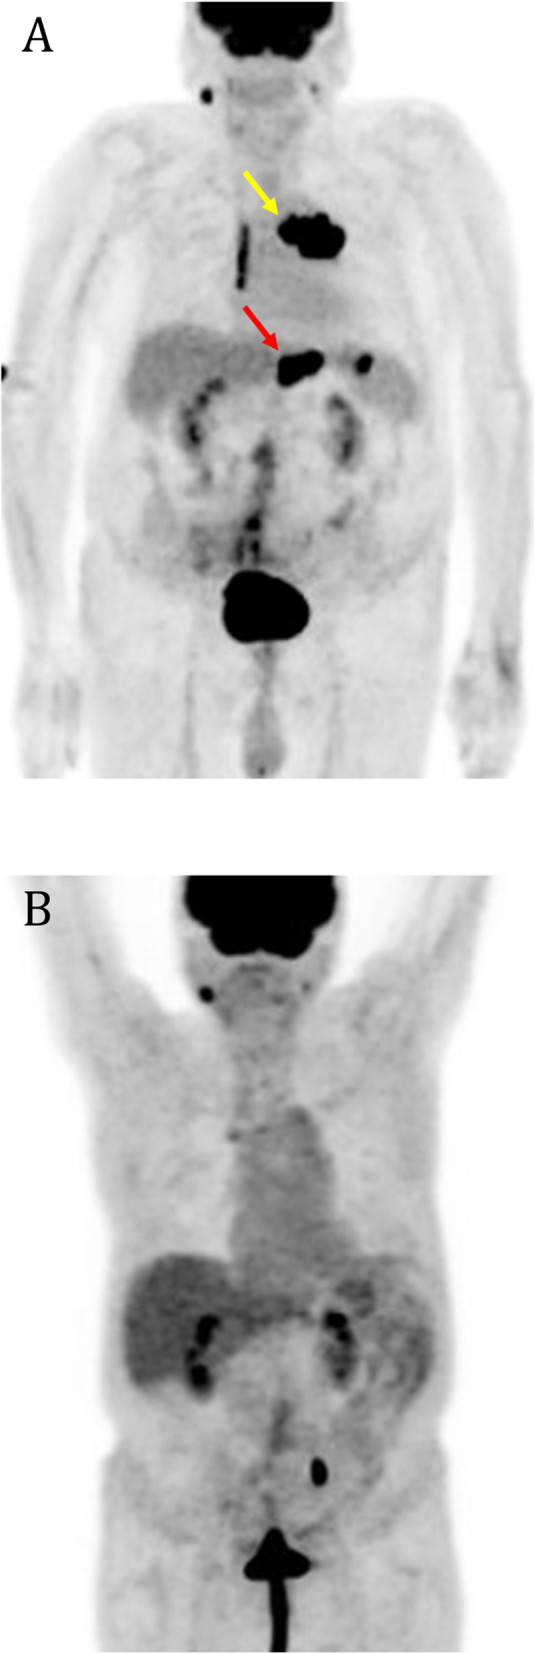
Positron emission tomography (PET) scan. a Pretreatment PET with active lymphoma on both sides of the diaphragm, including chest adenopathy and multifocal gastric involvement in the abdomen. *Yellow arrow*: chest adenopathy with standardized uptake value (SUV) of 20.4. *Red arrow*: gastric antrum uptake with SUV of 14.1. b Post-treatment PET about 6 months after resection showing no definite evidence of lymphoma
